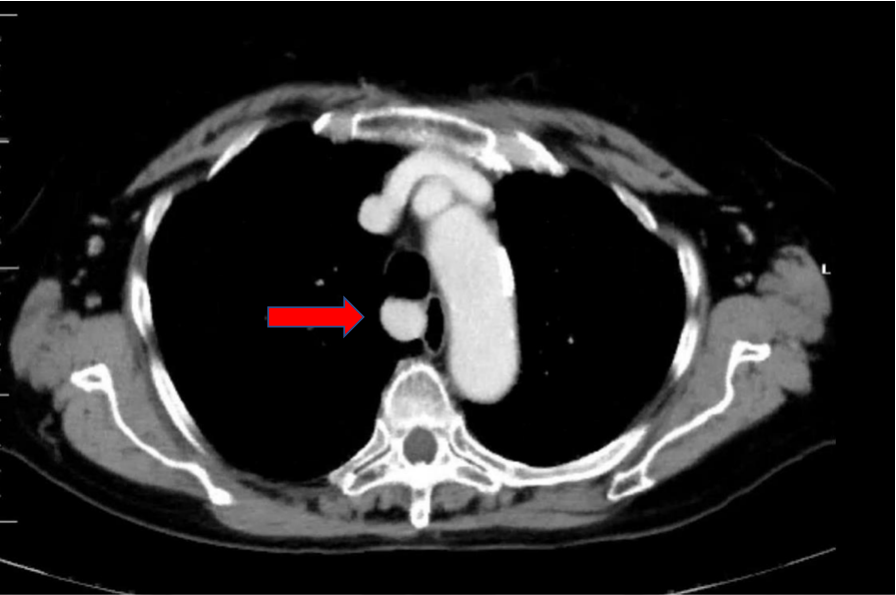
Chest computed tomography showing a small 17 × 16 mm nodule in the middle mediastinum
